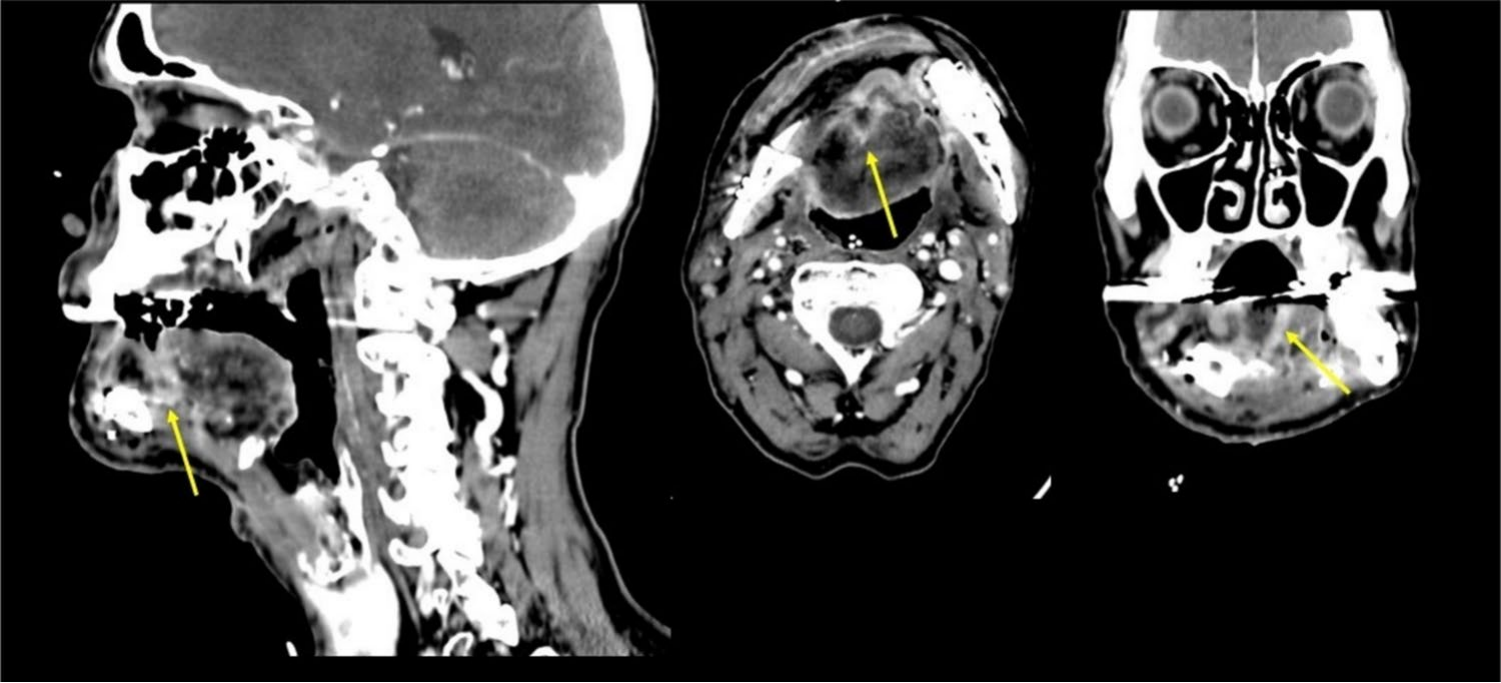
Sagittal, axial and coronal views of post-operative CE-CT after 5 weeks in a patient with pT4aN3b right inferior paramedian gingival SCC surgically treated with right emimandibulectomy, neck dissection and fibular osteo-cutaneous flap reconstruction. NI-RADS 2a for *T* supported by the presence of focal linear superficial post-contrast enhancement at the junction between the posterior margin of the adipo-cutaneous portion of the flap and the ventral tongue (yellow arrows)
